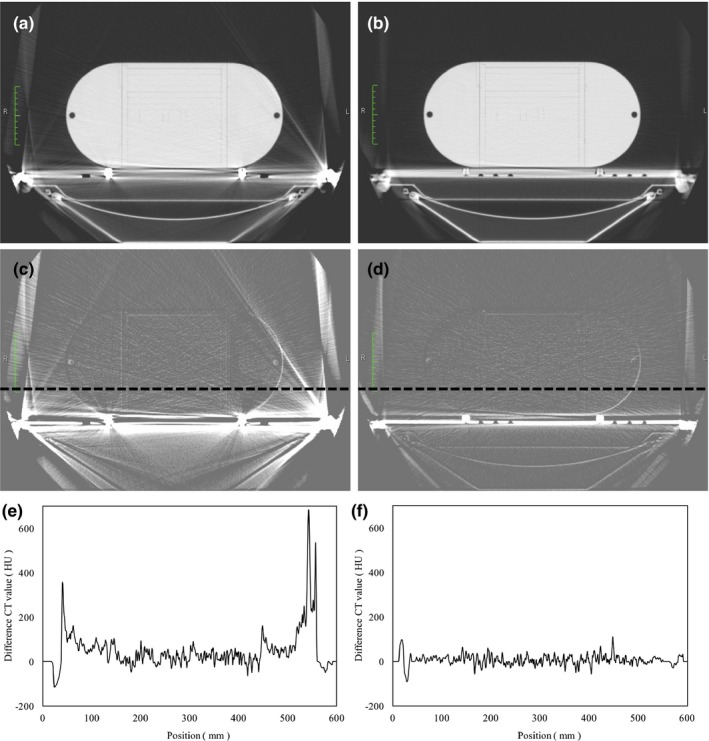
Artifacts produced by the lok‐bars. (a) VL‐bar. (b) HM‐bar. (c) VL‐bar; difference in CT image as a result of the presence of the lok‐bar. (d) HM‐bar; difference in the CT image as a result of the presence of the lok‐bar. (e) Profile curve for the CT values regarding the subtracted image of the VL‐bar (black dotted line) shown in (c). (f) Profile curve for the CT values regarding the subtracted image of the HM‐bar on the *X*‐axis (black dotted line in (d)).
